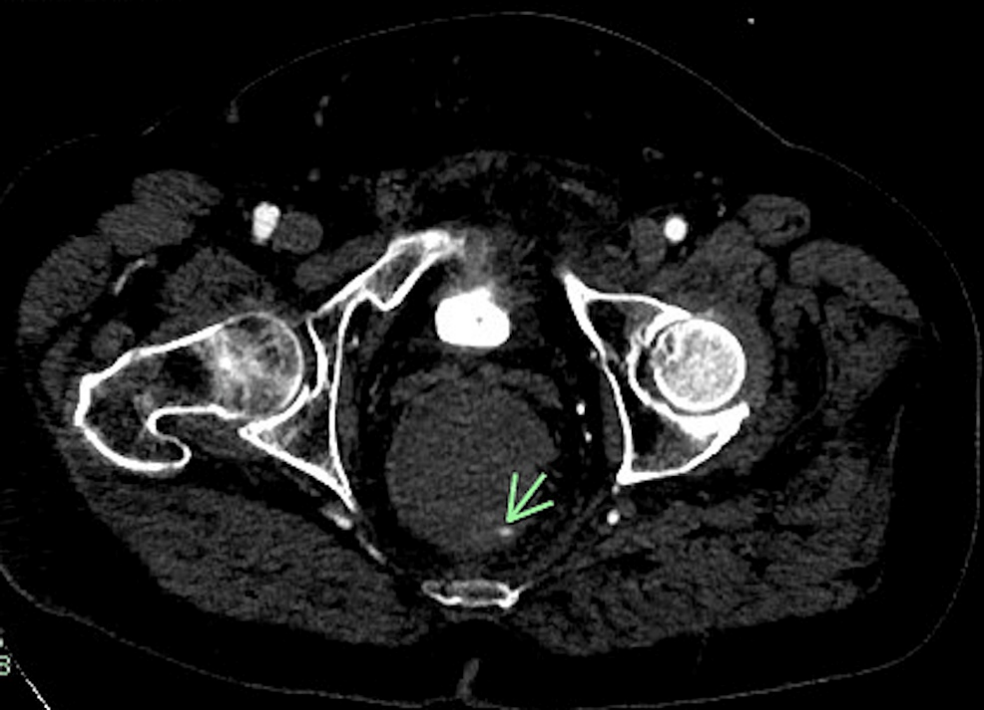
CT angiographic examination of the abdomen and pelvis demonstrating a focus of the intraluminal extravasation of the contrast along the left posterolateral aspect of the rectum.
